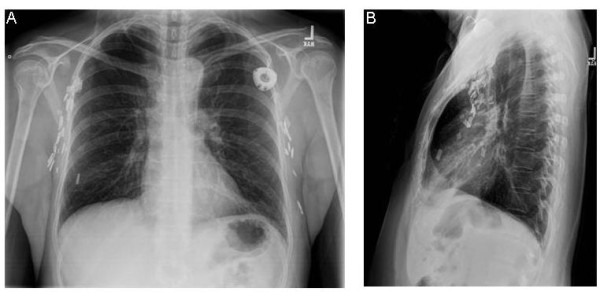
Posterioranterior (A) and lateral (B) chest x-ray views.
